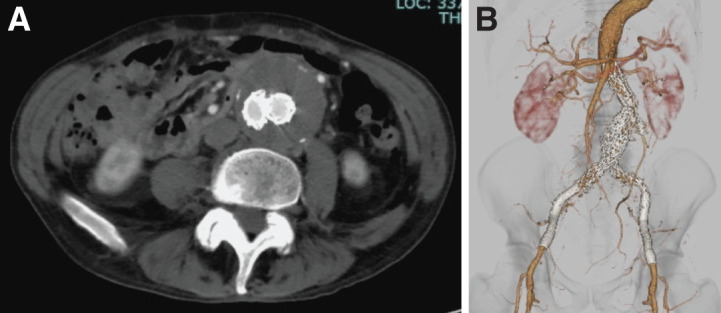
CTA images 4 days after EVAR. (A) Axial view of the aneurysm. (B) 3D virtual reality image. EVAR, endovascular aneurysm repair
